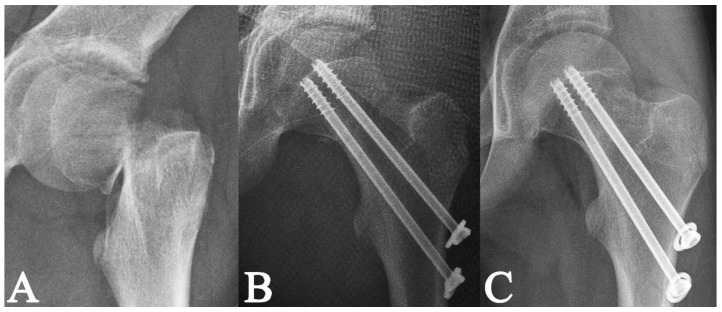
Radiographs of a 12-year-old boy with a displaced Delbet–Colonna type II fracture [15] (A); radiographs after open reduction and internal fixation with 2 cannulated ˄-shape screws; the fracture was completely compressed by the screws with the threads above the fracture line (B); the radiological healing at 2.8 months post-surgery (C).
